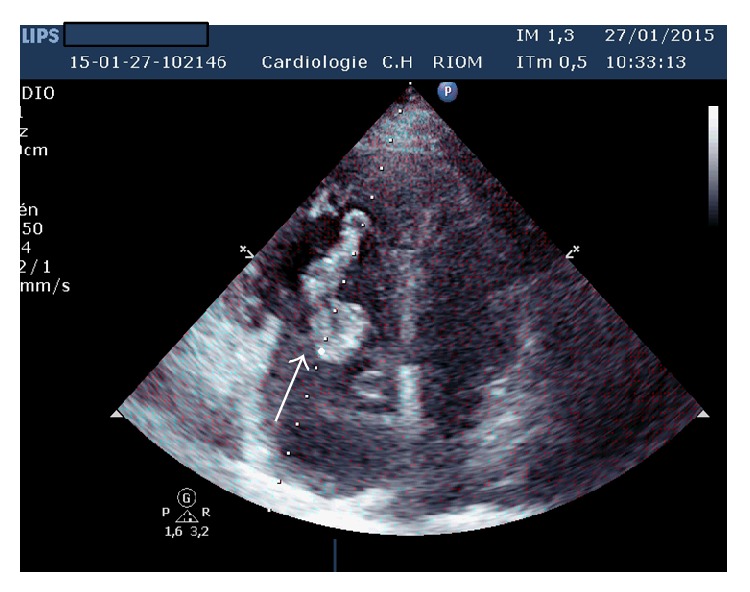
Apical four chamber view showing a highly mobile serpiginous thrombus in the right heart chambers (white arrow).
